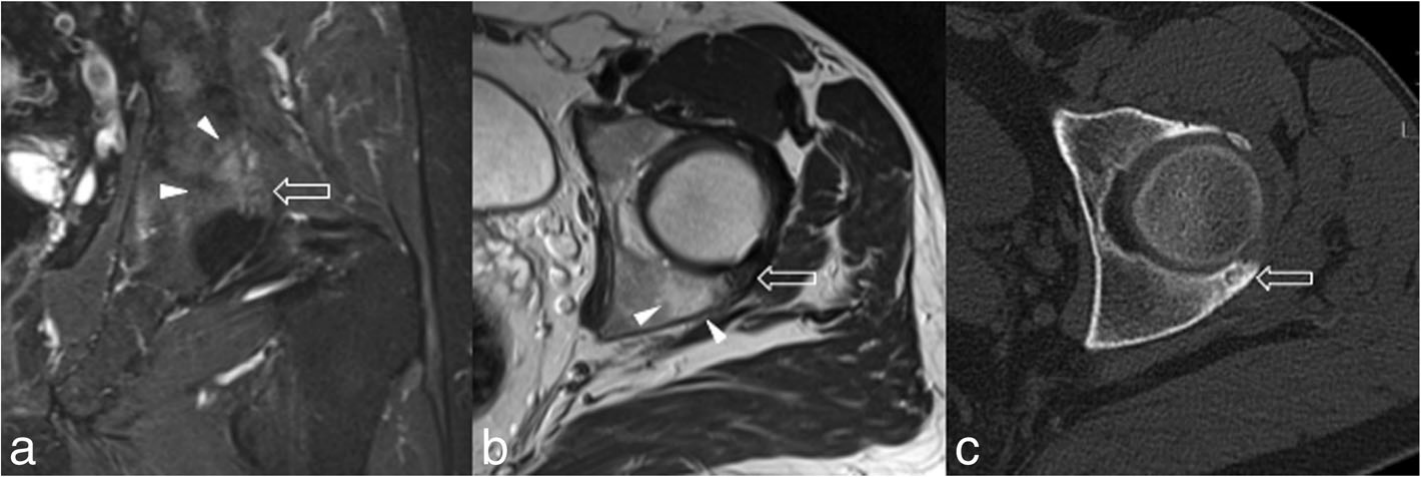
After 18 months, hip pain re-appeared, thus the patient underwent MRI (a, b) and CT (c) showing a recurrence of the previously treated OO of the posterior aspect of the acetabulum (void arrows), with extensive adjacent bone marrow oedema (arrowheads) well demonstrated on coronal fat-saturated proton density-weighted (a) and axial T2-weighted (b) images
